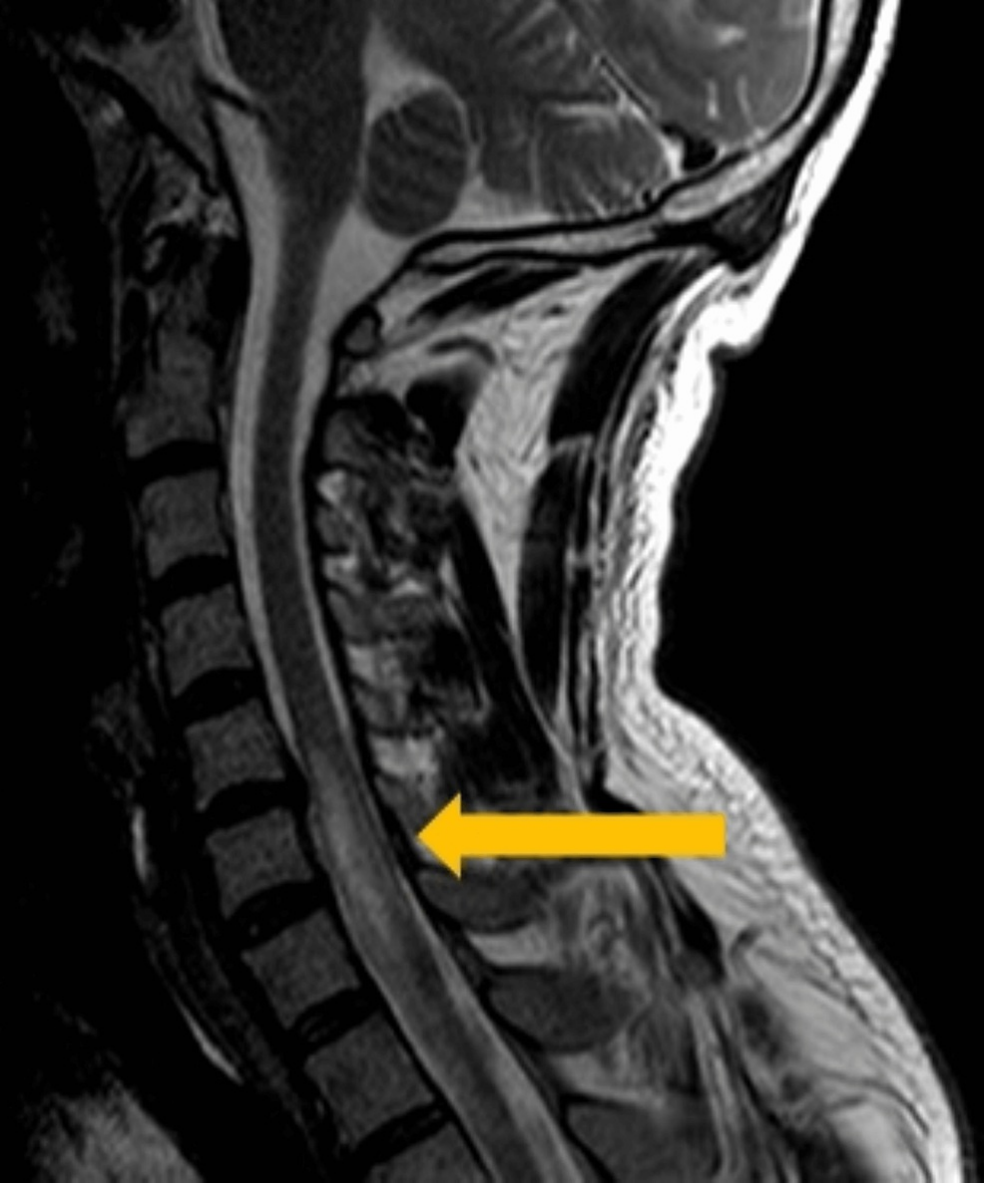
Magnetic resonance imaging showing a spinal cord infarction area on sagittal view. An arrow pointing to the spinal cord infarction area extending from C5 to T2.
